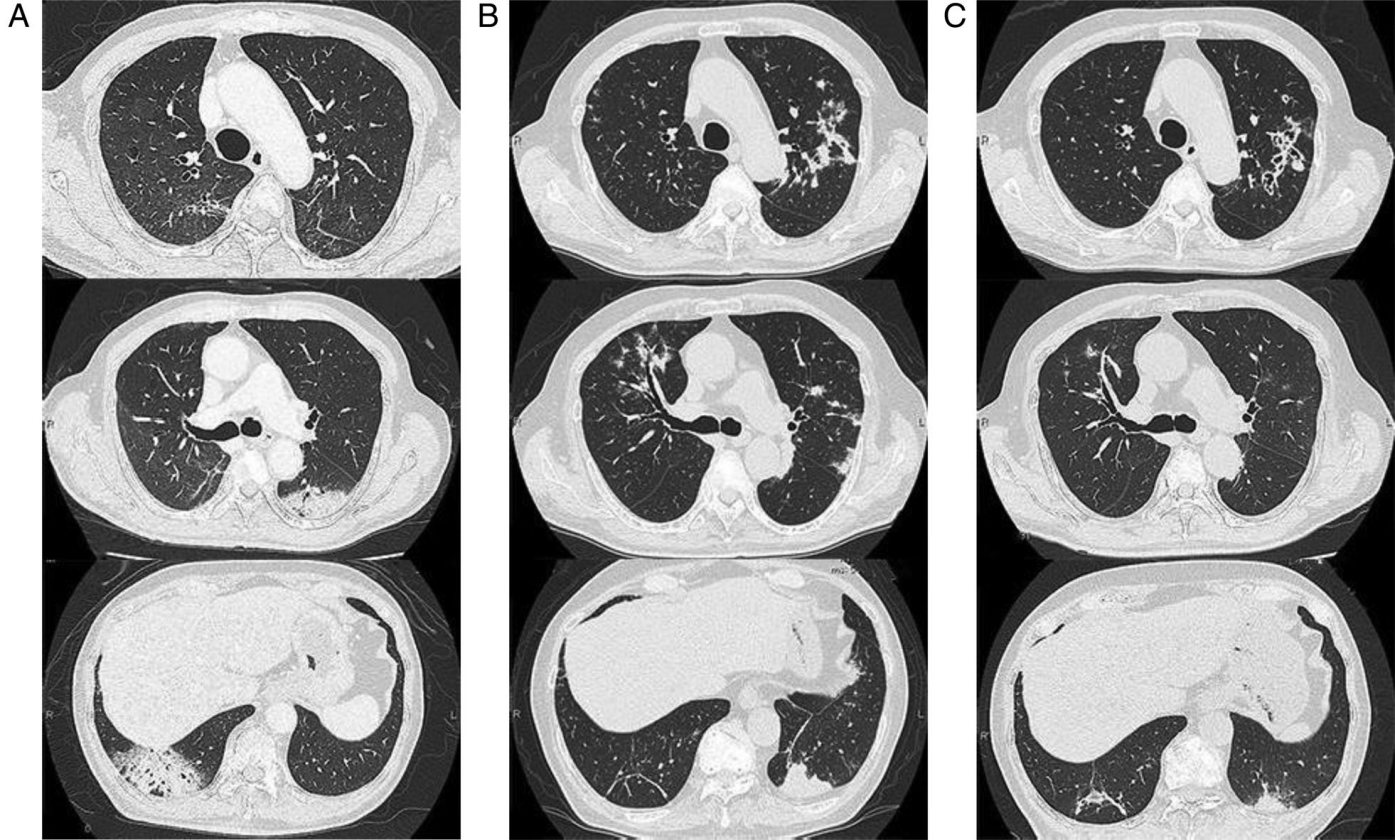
Radiological findings. (A) Chest computed tomography (CT) scan obtained at the time of the initial diagnosis of cryptogenic organizing pneumonia (COP). The corticosteroid had not yet been administered. Consolidations were observed on multiple lobes of the lung. No nodular opacities were observed in the lung. (B) Chest CT scan obtained at the first visit to our hospital showing consolidation of the left lower lobe and multiple nodular shadows with thickened bronchial walls in the lungs bilaterally. Initial consolidations observed at the time of the diagnosis of COP had disappeared. (C) Chest CT scan obtained after taking fluconazole for six months. Consolidations of the left lower lobe had shrunk, and multiple nodular shadows had reduced. Bronchiectasis is observed in the left upper lobe.
